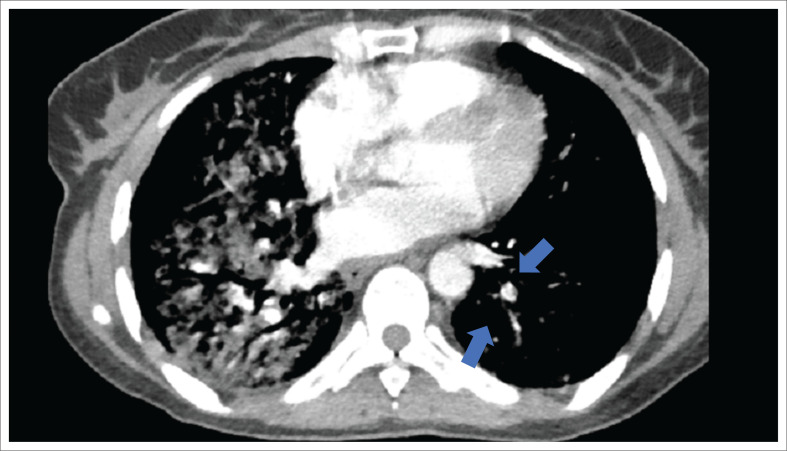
Computed tomography pulmonary angiogram showing filling defect in left lower lobe subsegmental pulmonary artery.
